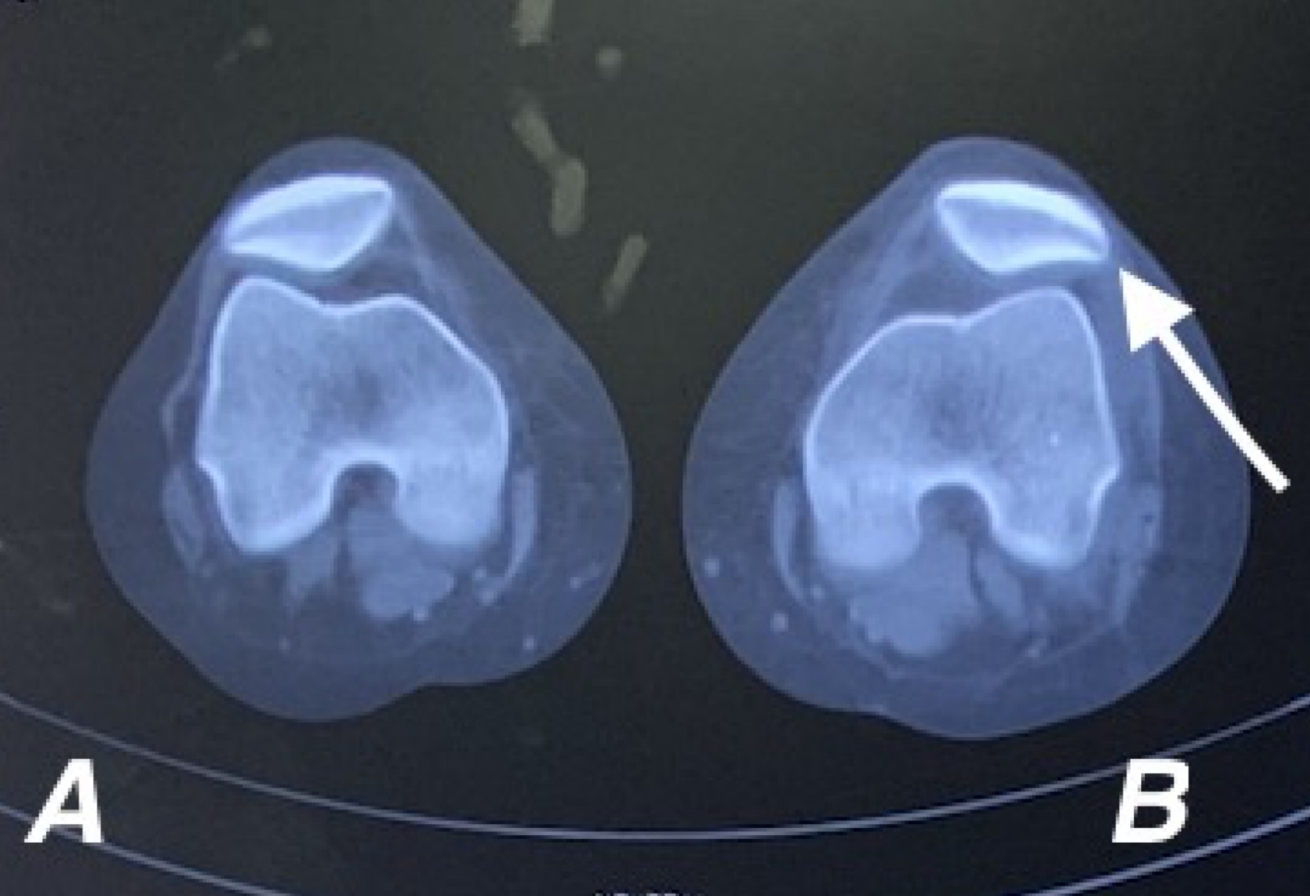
Axial CT cuts of A) right and B) left knees showing left lateral patellar tracking.
